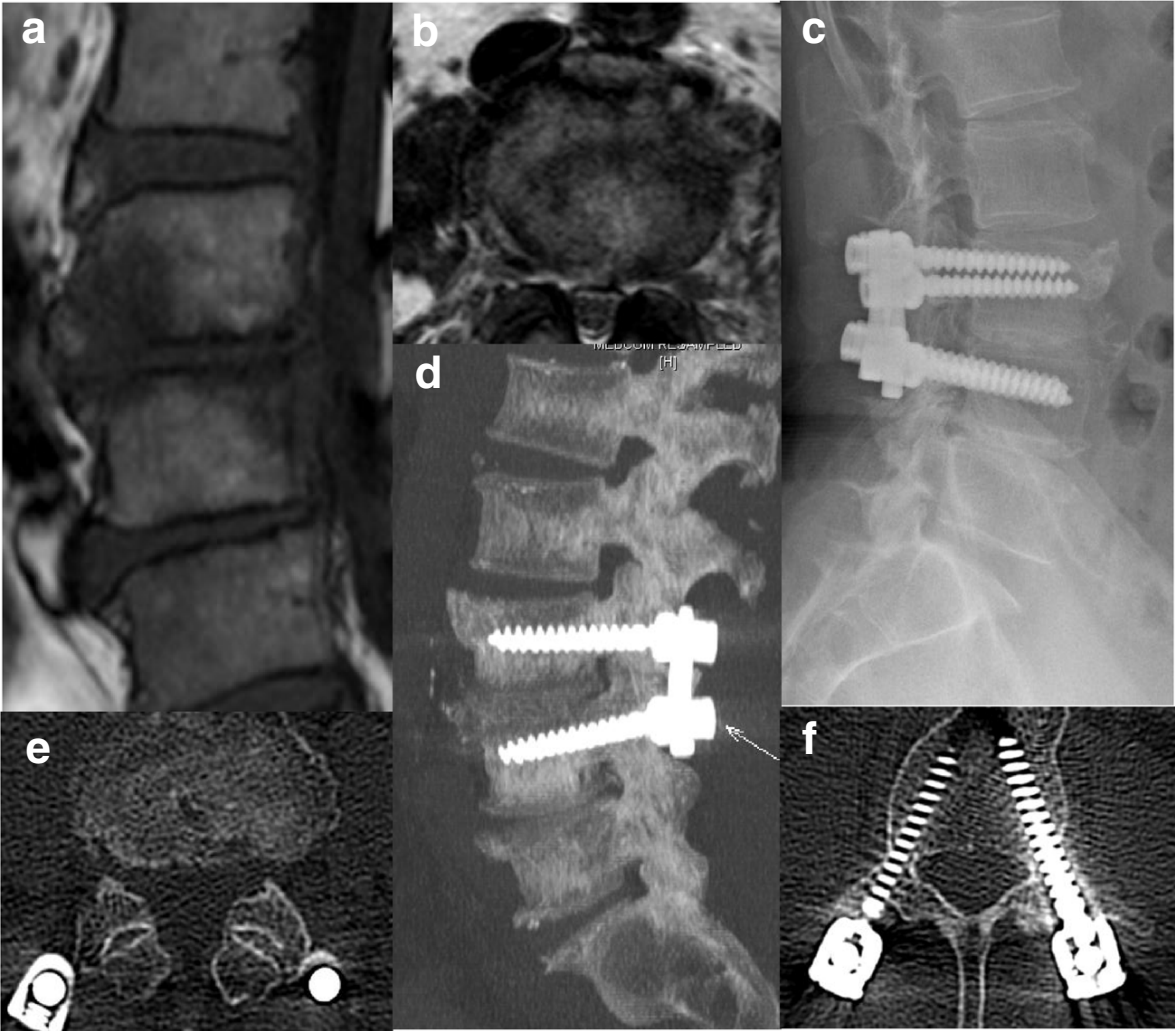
Exemplary case of a 61-year-old female presenting with immobilizing low back pain (VAS 6/10), fever and elevated procalcitonin, ESR, and CRP level. Sagittal (a) T1-weighted showed abscess in the frontal part of L3/4 disc space and axial (b) T2-weighted MR images demonstrated bony endplate lesion and purulent mass at the same level. Post-operative X-ray at 12 months (c) and spine computed tomography demonstrated bony fusion on the sagittal view at 30 months post-operatively (d). The canal was adequately debrided and decompressed on the axial view (e) with no screw loosening (f)
